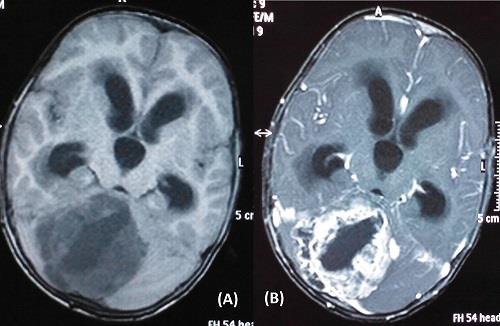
Non-contrast (A) and contrast enhanced (B)T1-weighted magnetic resonance images of a patient showing solid-cystic mass with heterogeneous contrast enhancement and intensity sugges-tive of medulloblastoma.
